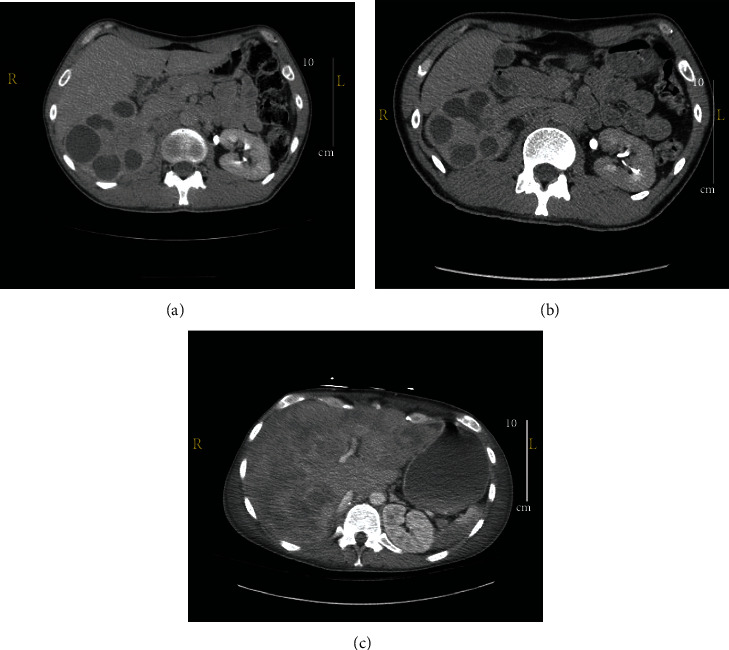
Radiographic findings. (a) Contrast-enhanced CT abdomen on initial presentation demonstrating an enlarged right kidney with hydronephrosis and perinephric stranding which is consistent with the “bear claw sign” that suggests XGP. (b) Contrast-enhanced CT abdomen prior to nephrectomy demonstrating aggressive progression of right kidney disease with invasion of surrounding structures. (c) Contrast-enhanced CT abdomen demonstrating multiple biopsy-confirmed metastatic liver lesions from urothelial carcinoma.
